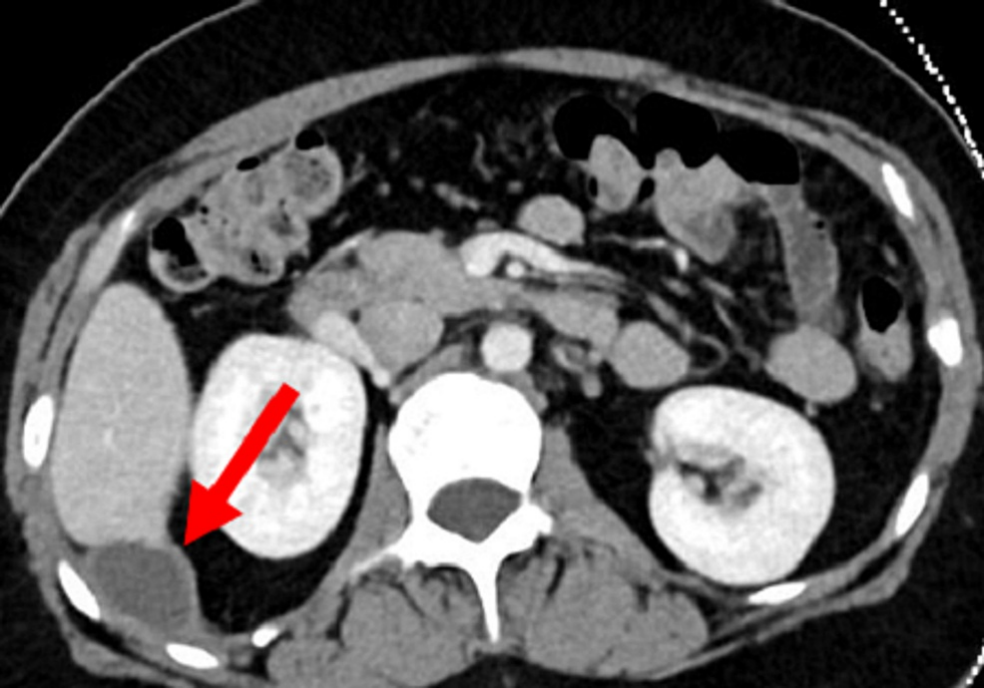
A transverse view of the CT scan showing a subdiaphragmatic collection (red arrow). CT: Computed tomography.
